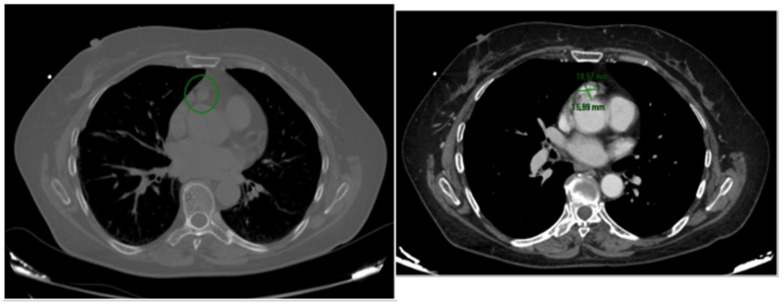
A CT scan with contrast was ordered.
